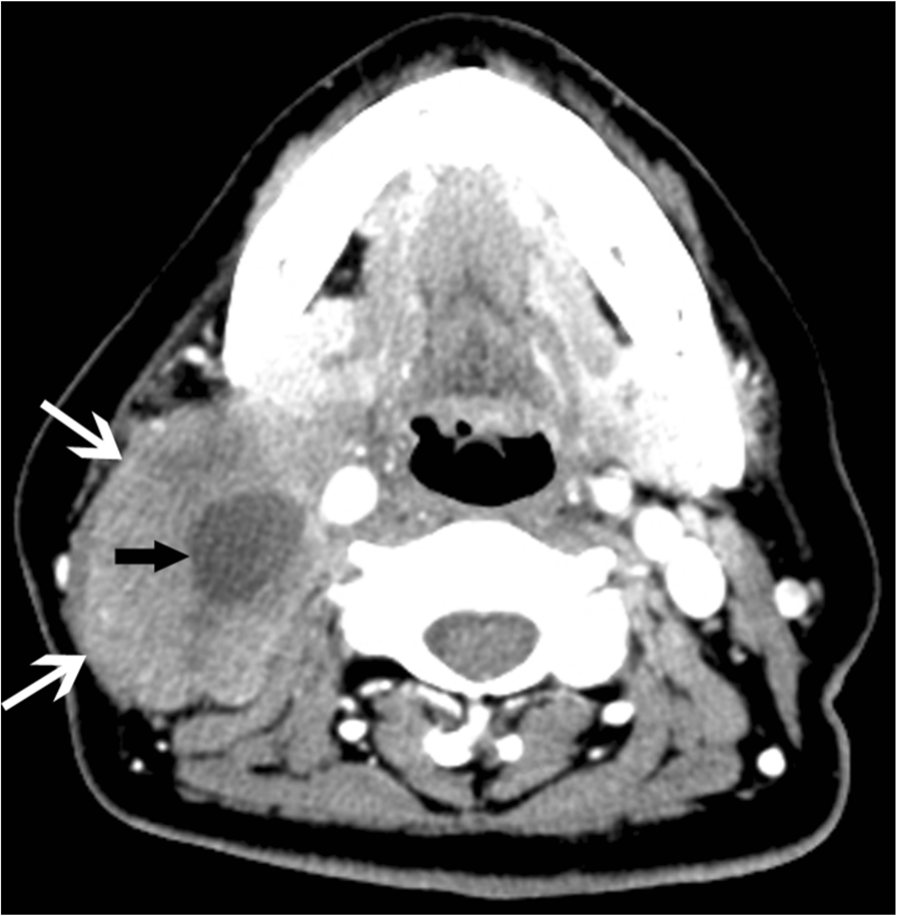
A 47-year-old woman who presented with non-Hodgkin’s lymphoma. A contrast-enhanced CT image of the neck shows an ill-defined margin (white arrow) and a region of liquefaction and necrosis with a mild enhancement pattern in the interior of the mass (black arrow)
